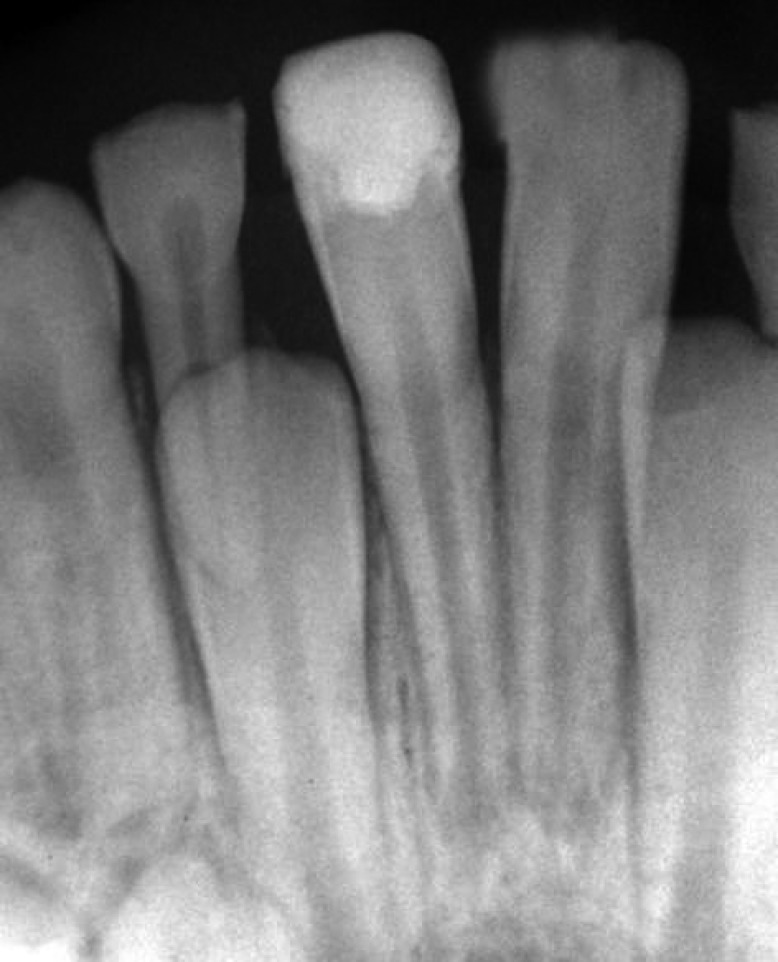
Radiographic image of the central mandibular incisors 6 months after treatment
